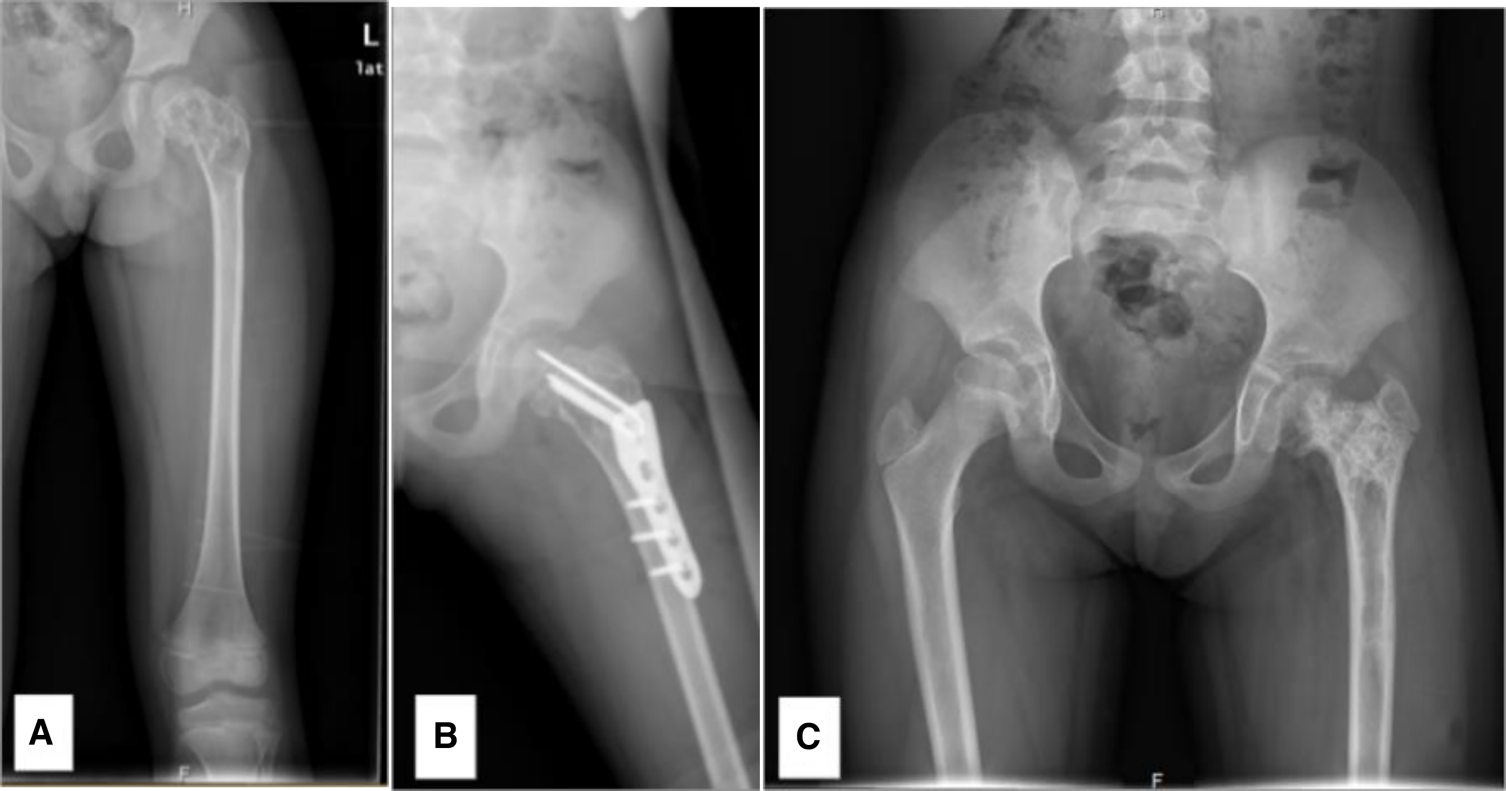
A 6.76-year-old female diagnosed with a SBC in the proximal left femur, combined with a fracture. (A) Preoperative radiograph. (B) Radiograph taken 3 days post-biopsy, curettage, LCP-PHP procedure, and bone grafting. (C) Radiograph at 1.9 years post-surgery, revealing premature closure of the epiphysis, shortening of the femoral neck, and coxa vara.
